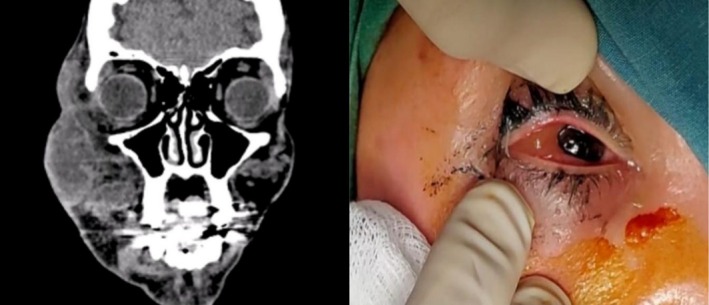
Left picture of a CT conducted and showing soft tissue thickening and fat stranding involving the right side of the face including the temporal region and orbit highly suggestive of cellulitis and orbital cellulitis. Right picture showing the intraoperative findings of the case.
